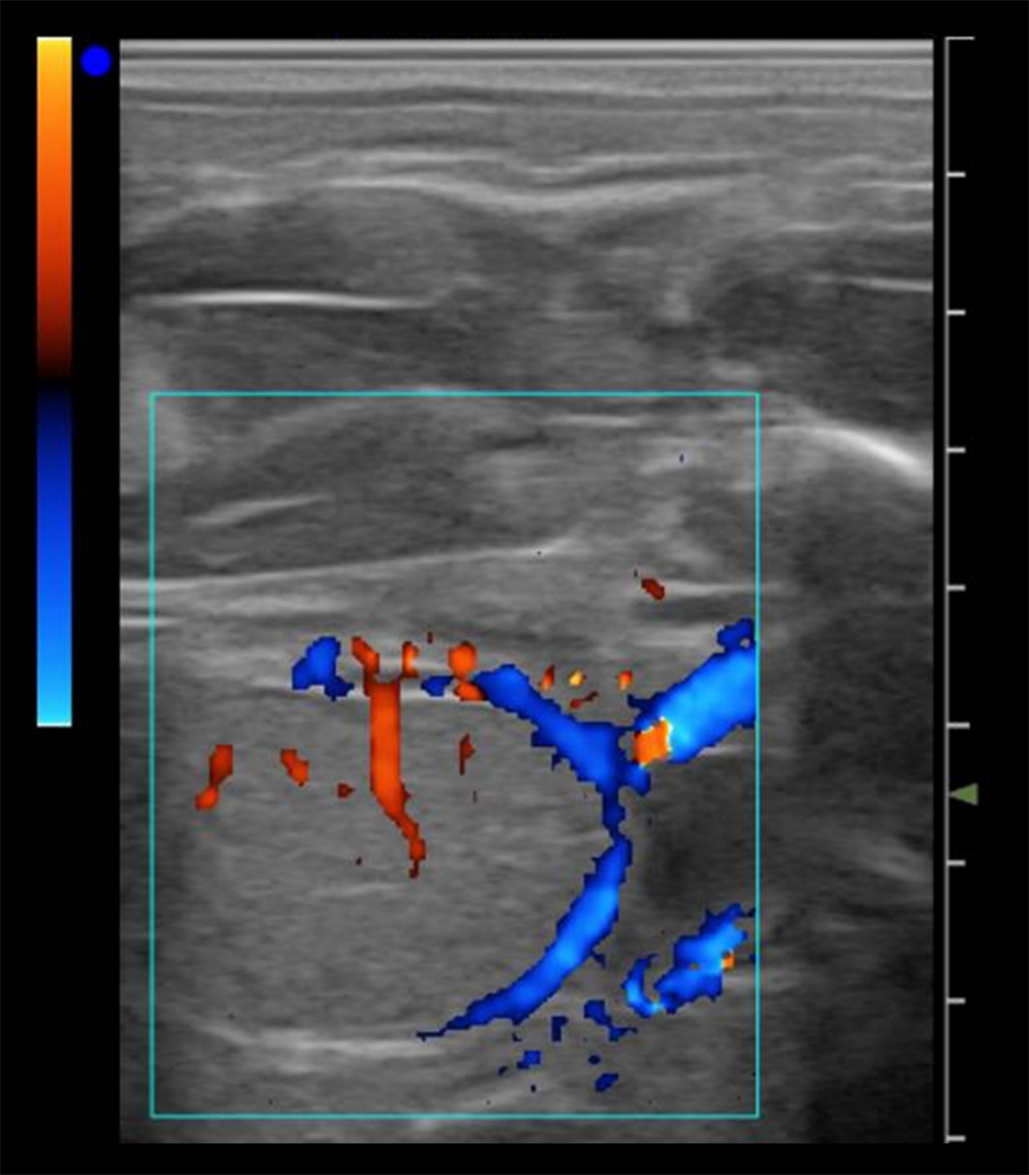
Determination of fetal sex by Doppler ultrasound. Colour Doppler ultrasound image at day 240 of gestation, showing the vascularization pattern characteristic of the male gonad, with the pampiniform plexus and the testicular vein
